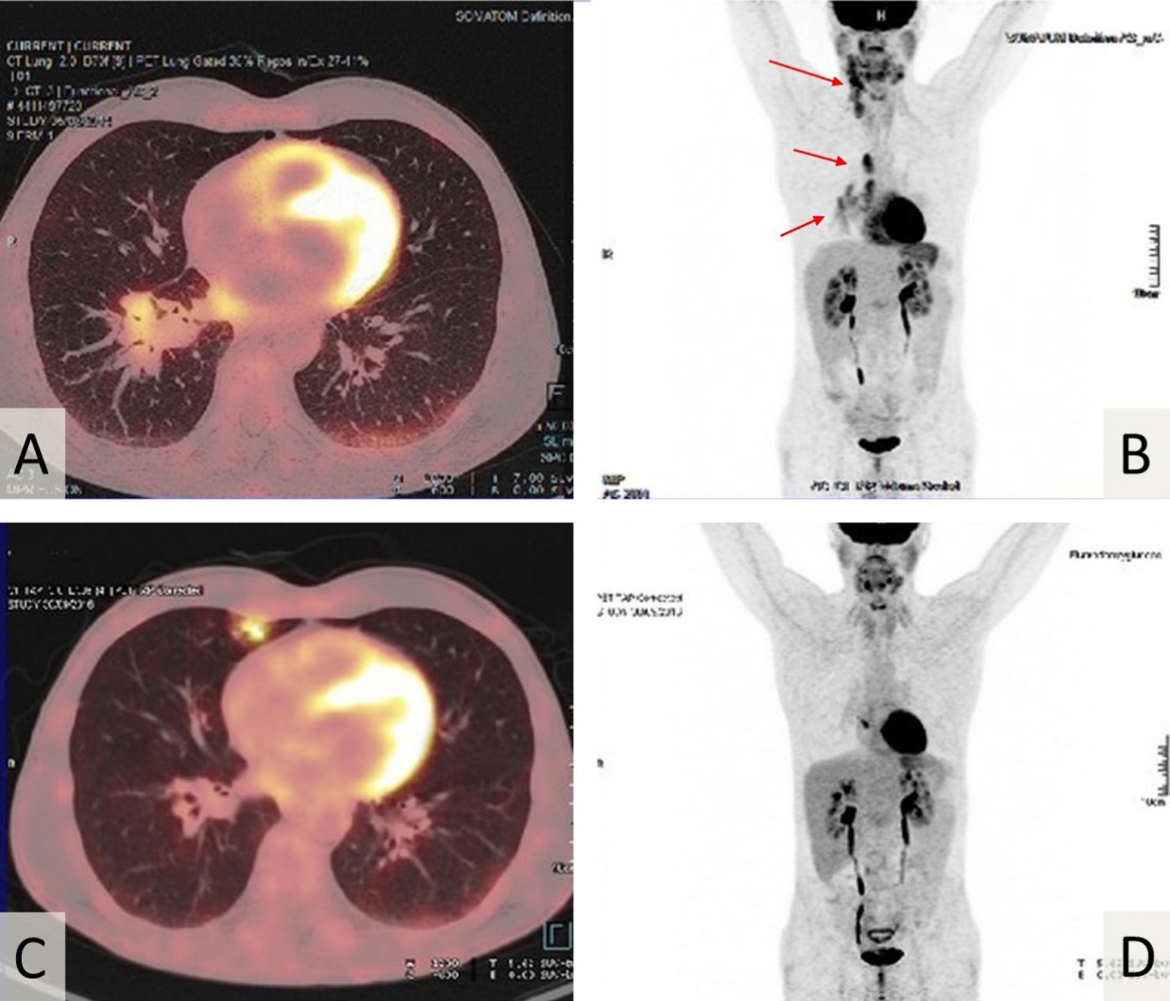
PET-scan imaging. A, B PET-scan of July 2014 at time of diagnosis, showing hypermetabolic cervical, mediastinal and hilar lymphadenopathies (red arrows). There was no digestive localization. C, D PET-scan of October 2016, showing pulmonary FDG uptake compatible with lung infection. No suspect FDG uptake of lymphoma recurrence or digestive uptake was found
